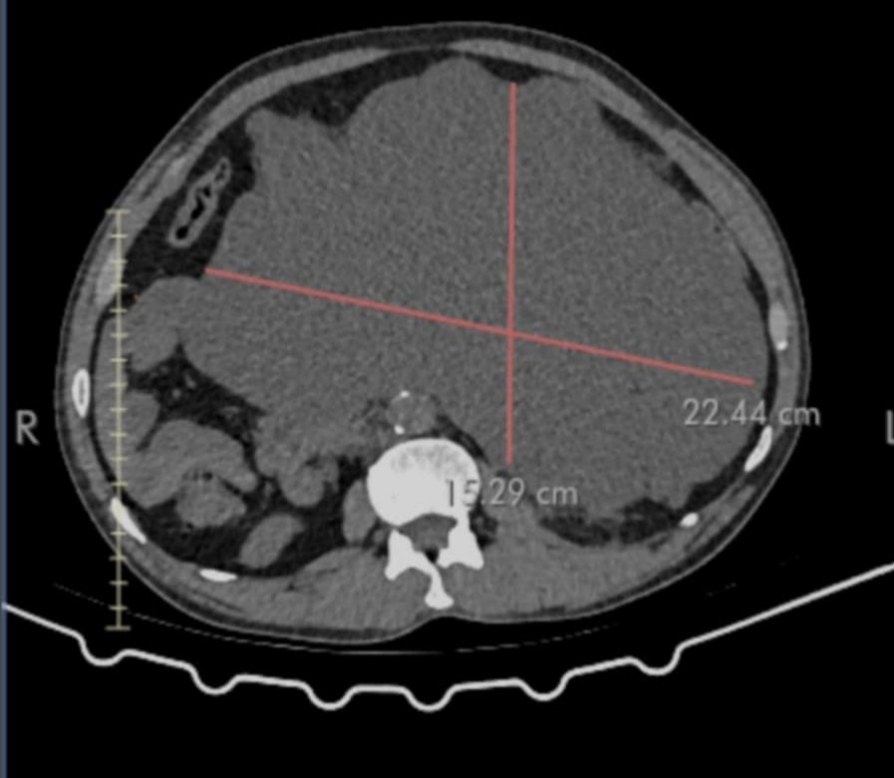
A computed tomography scan of the abdomen and pelvis revealed a larger, heterogeneous well-defined mass (22.44 × 15.29 cm) in the mesenteric region.
